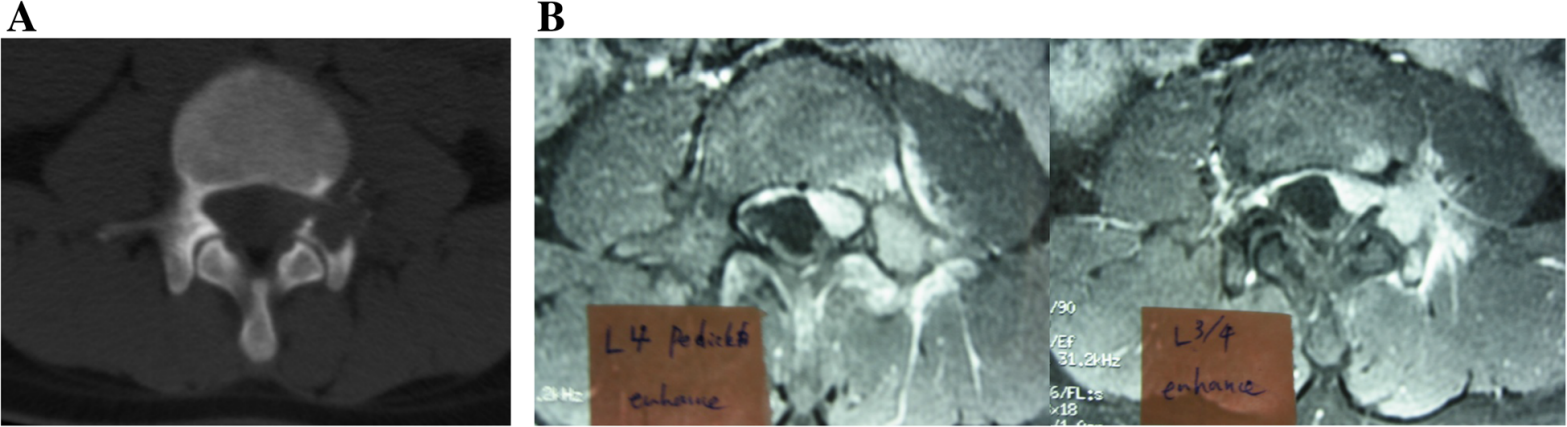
a Axial CT of L4 (performed at a previous hospital) showed an osteolytic lesion around the left pedicle. The cortex was seen to be broken by the tumor. b T1 axial MRI of the L4 and L3/4 level with Gd enhancement. The left pedicle and its surrounding tissues show enhancement, while the tumor extends to the epidural space
